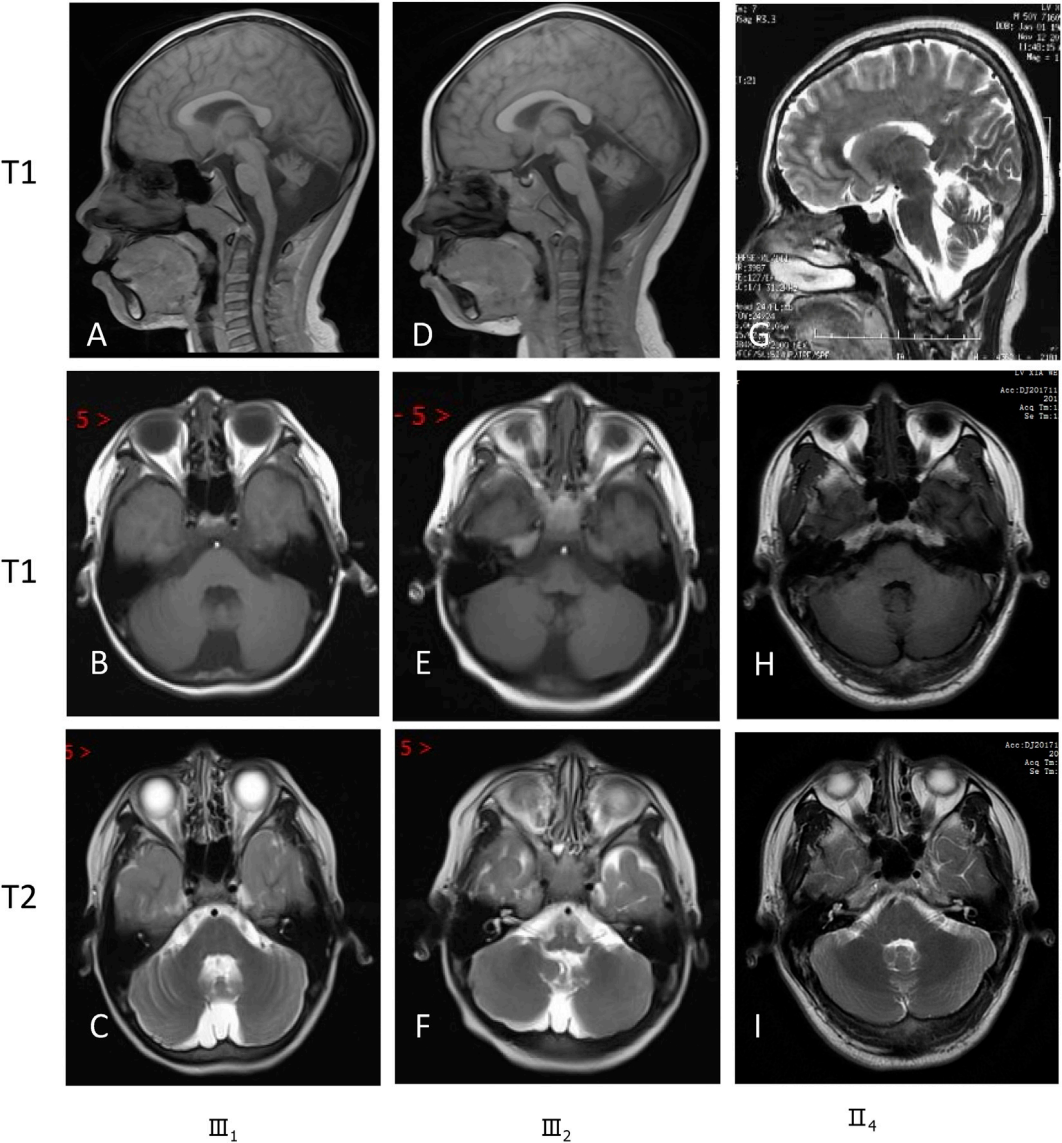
Magnetic resonance images show severe hypoplasia of the cerebellum (III1 and III2).
